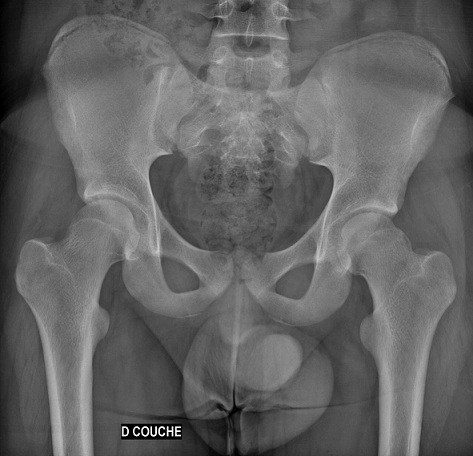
Radiographie montrant la réduction de la luxation de la hanche gauche
